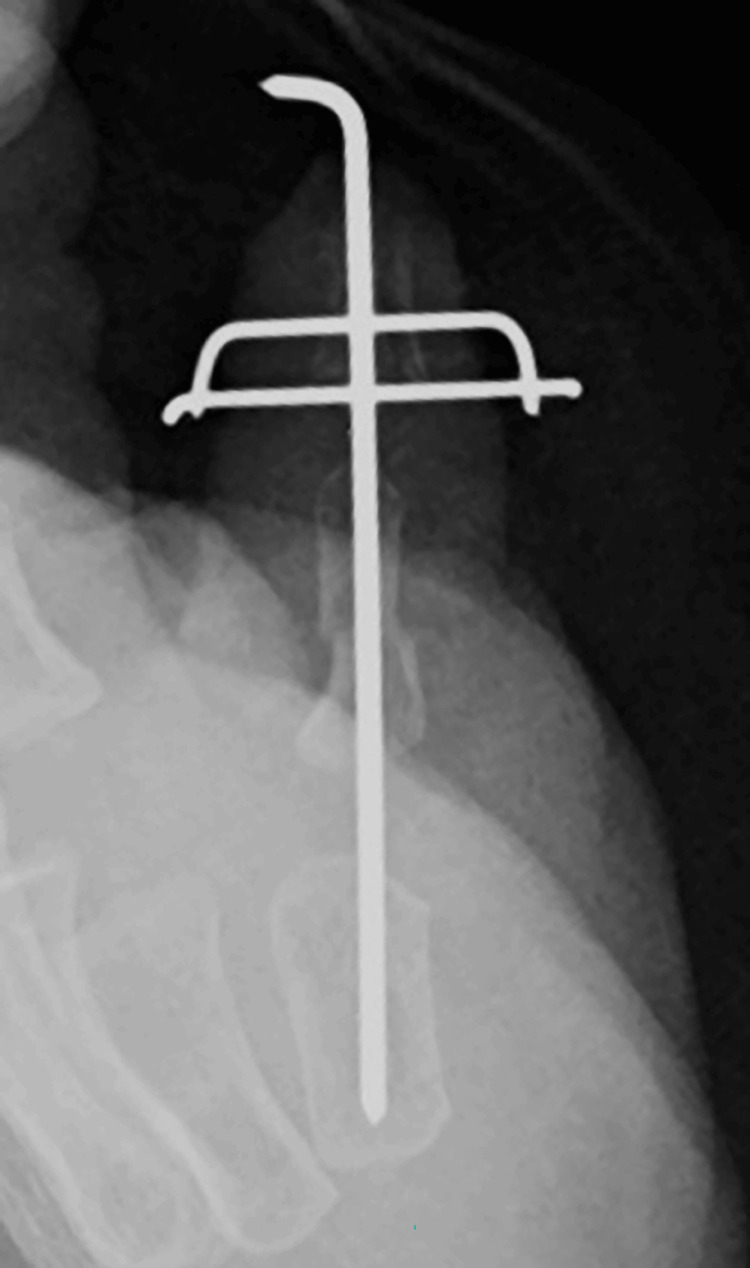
Radiographic image after the surgery
